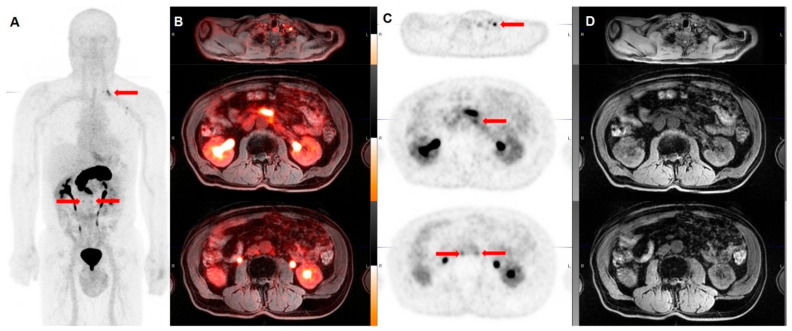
Whole body Maximum Intensity Projection (MIP, A), fused coronal ^68^Ga-RM2-PET/MRI (B), coronal emissive ^68^Ga-RM2-PET (C), and MRI images (D) in a 74 year-old man with PCa in biochemical recurrence (PSA 8.4 ng/mL). Focal 68Ga-RM2 uptake is seen in left supraclavicular and retroperitoneal lymph nodes (red arrows). (Images courtesy of Andrei Iagaru, MD, Stanford University).
